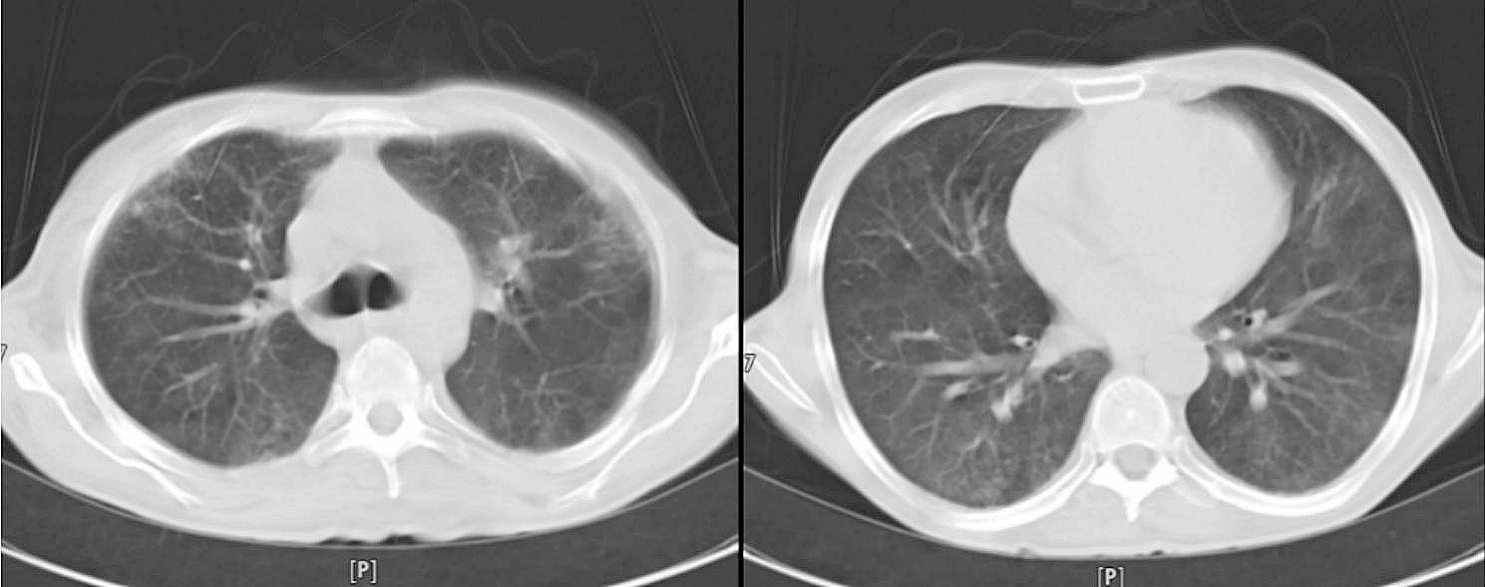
Spiral chest CT scan of the case 1, 06/28/2022. The Fig. 2 concludes his test outcome generally. Radiographic abnormality improved dramatically
